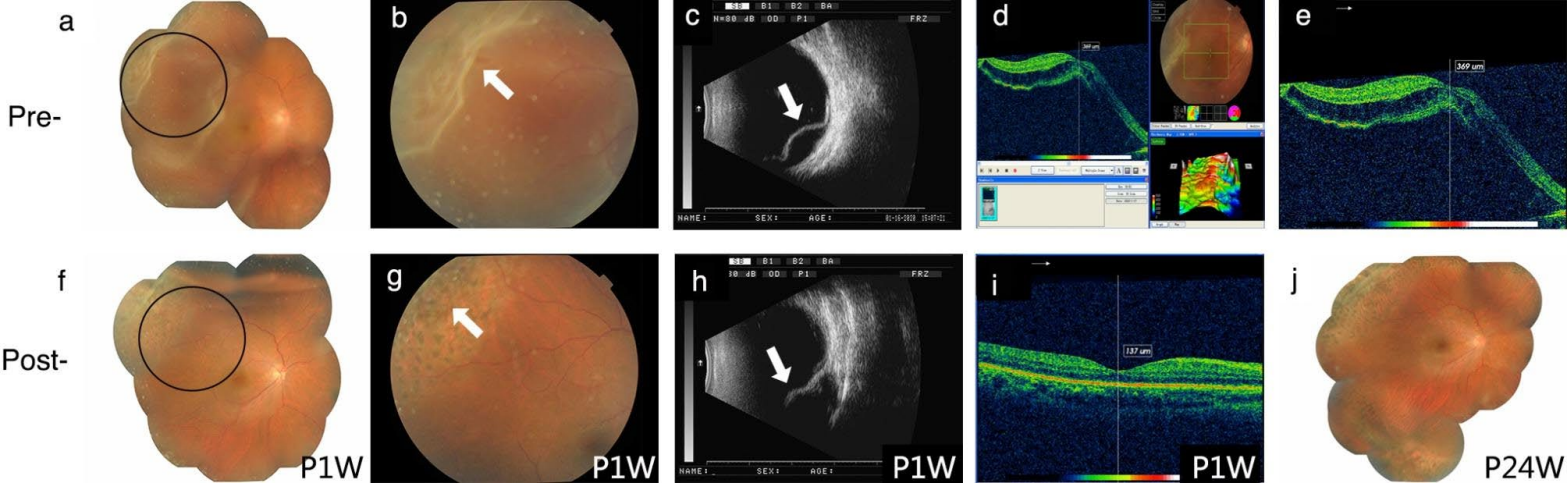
FCB scleral buckling efficacy on patient 1 (a) Fundus image showing retinal detachment pre-surgery. (b) The amplified circle area from A shows the retinal tear (white arrow). (c) B-ultrasound scan showing retinal detachment pre-surgery (white arrow). d–e. OCT shows an unaffected macula. f. Fundus image of FCB scleral buckling at 1-week post-surgery (P1W). g. Fundus image showing clear and distinct photocoagulation spots (white arrow). h. Post-operative B-ultrasound scan showing FCB’s evident pressure on the sclera (white arrow). i. OCT shows a small amount of remaining subretinal fluid and the mostly reattached retina. j. Fundus image at 24 weeks post-operation (P24W) showing the well-reattached retina
